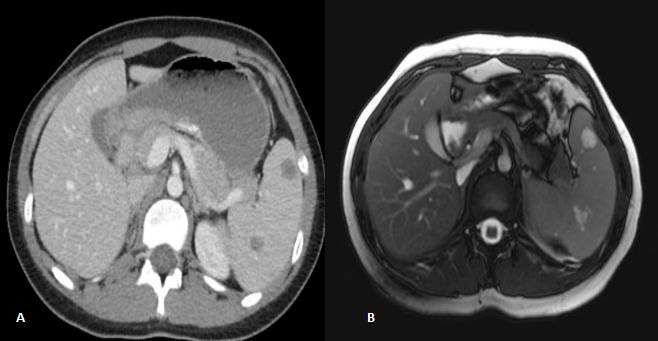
Figure 1:Computerized tomography (A) and magnetic resonance imaging (B) of the patient.
